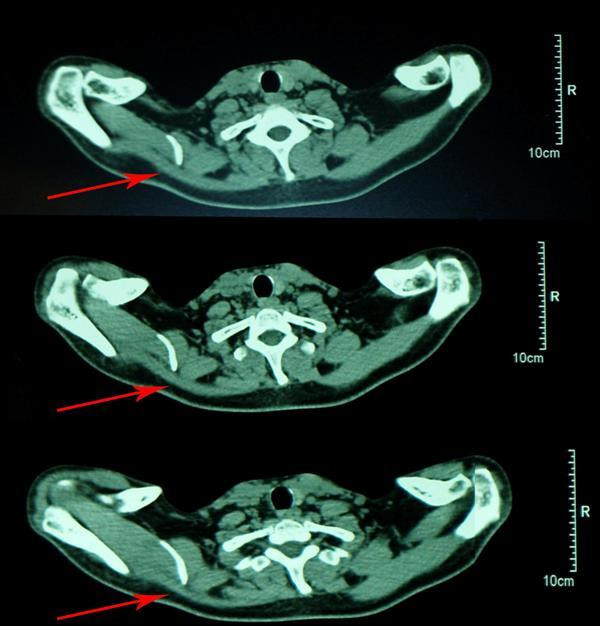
Transverse views of computed tomography scans reveal marked wasting in the muscle bulk of almost all parts of the right trapezius (arrows).
